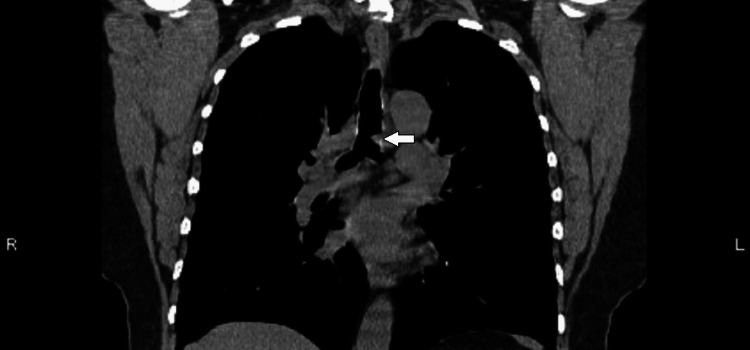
Coronal CT image with a white arrow indicating a solitary endotracheal polypoid mass near the bronchial bifurcation
